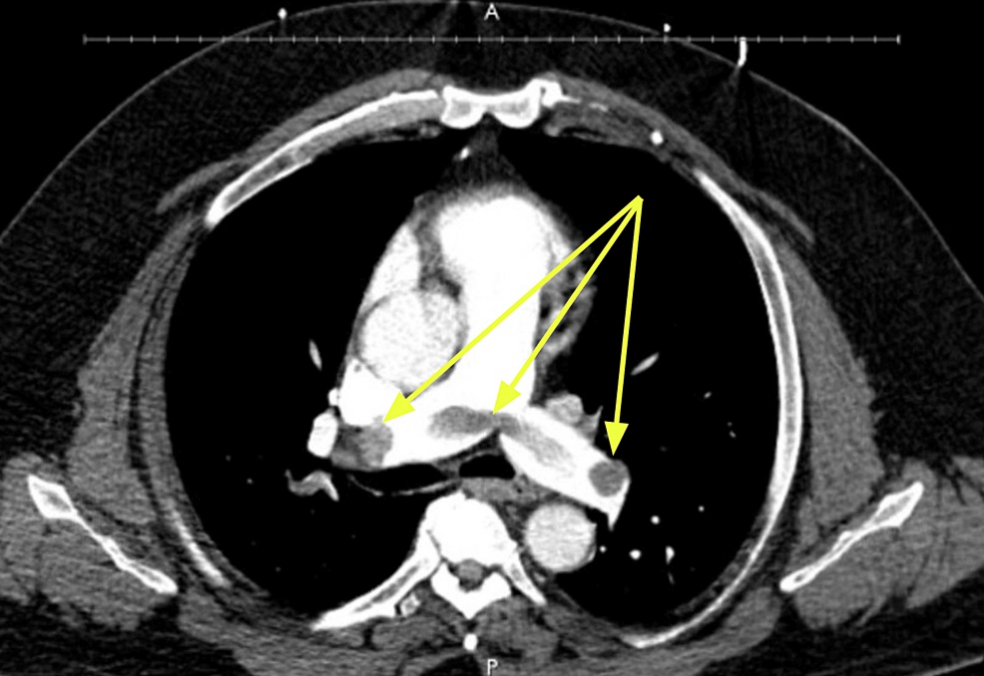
Chest computed tomography angiography Arrows pointing toward hypodensities within the bilateral pulmonary arteries are indicative of a bilateral pulmonary embolism
